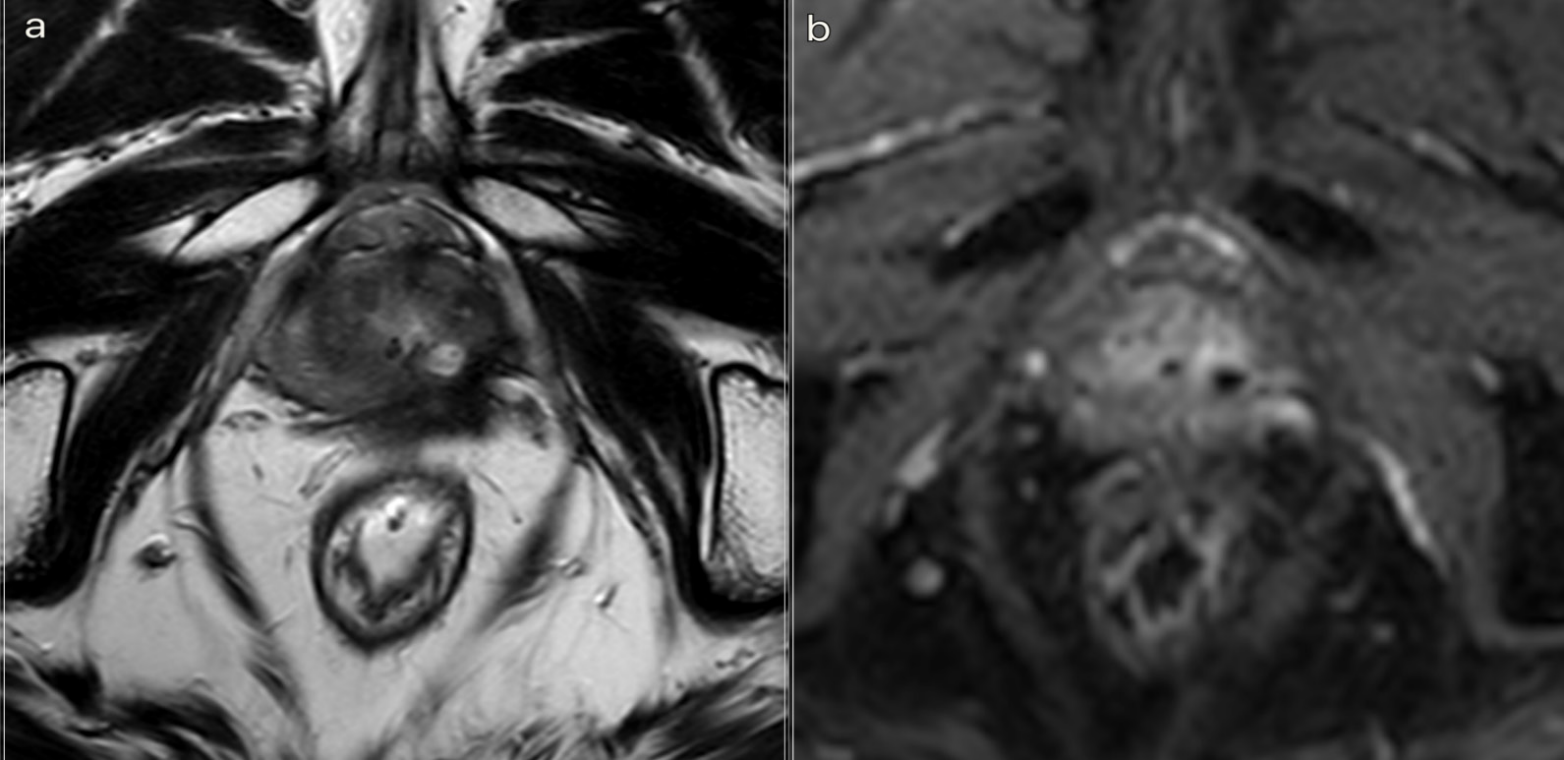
3T multiparametric MRI performed at 18 month follow-up. (a) *T*
